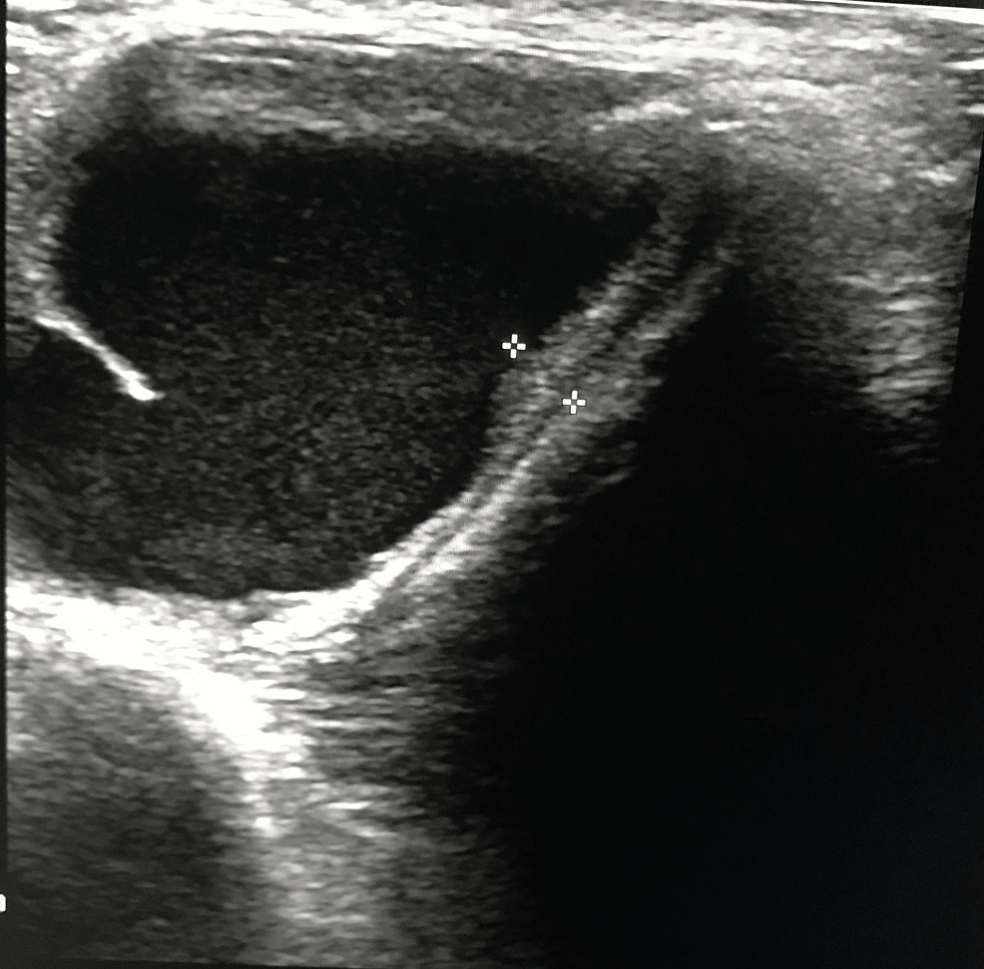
Ultrasonography showing thyroglossal cyst with tract.
